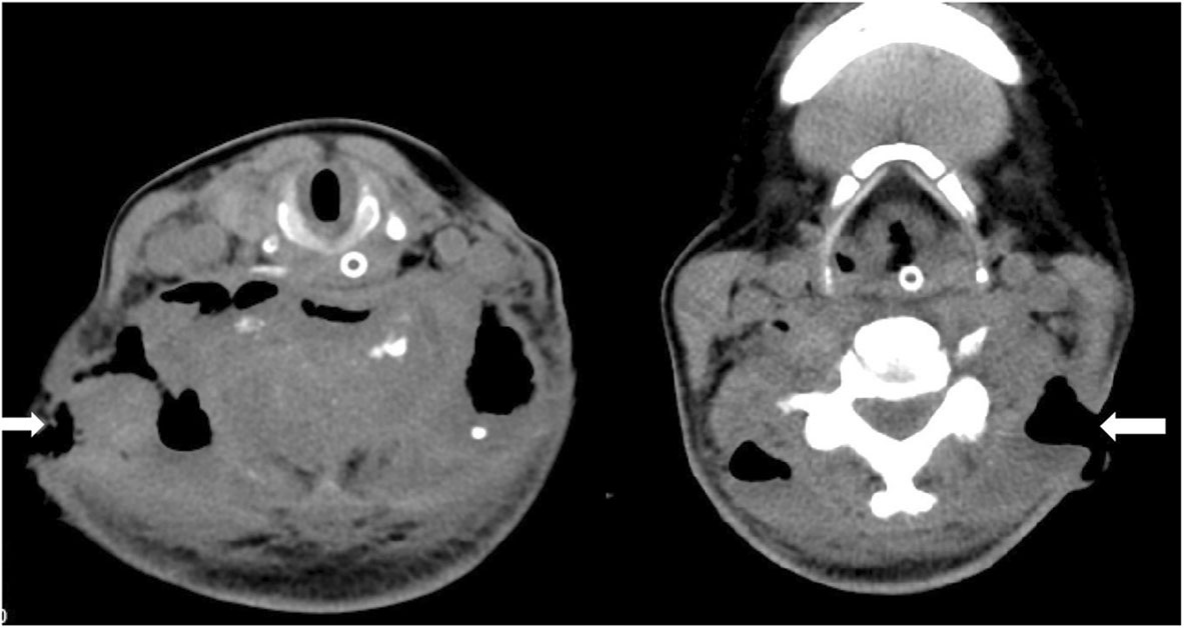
Cervical CT presented bilateral prevertebral abscesses, subcutaneous emphysema and skin fistulas (demonstrated by the arrows)
